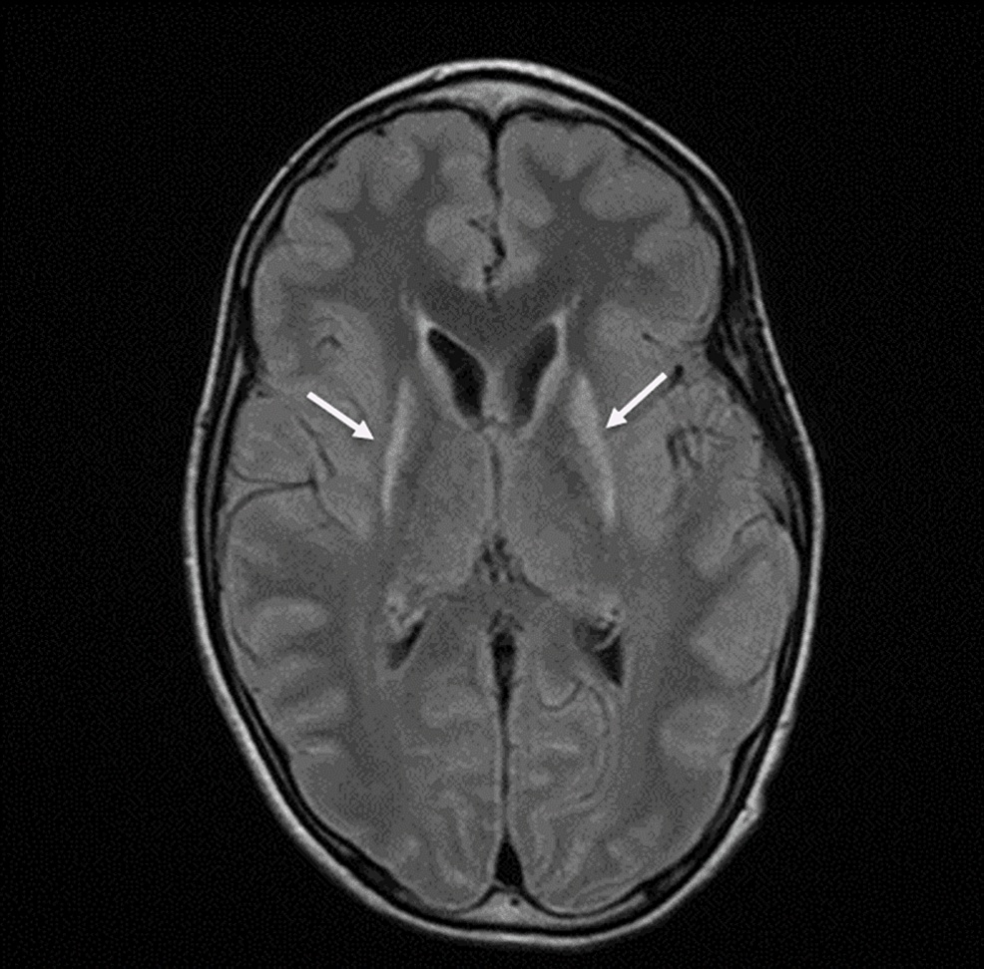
Axial FLAIR MRI of the brain shows symmetric areas of abnormal T2 hyperintensity involving the bilateral putamen (white arrows).
